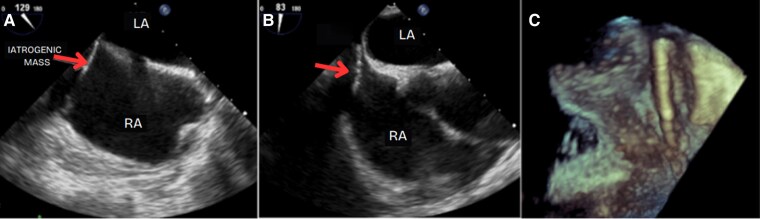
A transoesophageal echocardiography showing an elongated, rigid accessory mass, likely of iatrogenic origin, extending from the right atrium (RA) into the right ventricle in two-dimensional and three-dimensional images. LA, left atrium.
